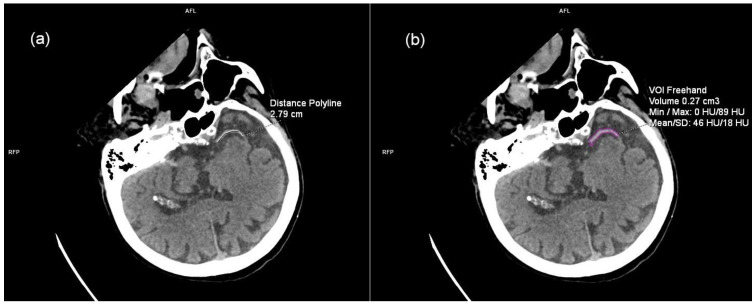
Thrombus characteristics measured on unenhanced brain CT after multiplanar positioning to show the full extent of the hyperdensity. (a) Thrombus length measured by distance polyline. (b) Thrombus volume and density measured by free-hand VOI. VOI = volume of interest; HU = Hounsfield units; SD = standard deviation.
